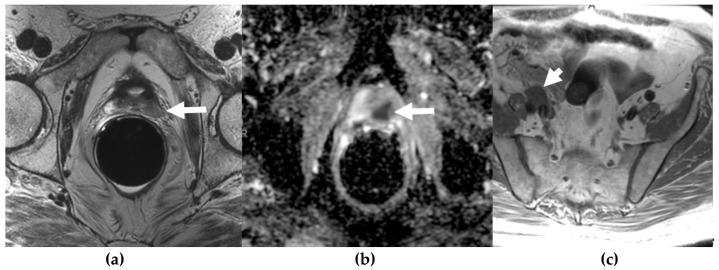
A 60-year-old man with lower retroperitoneal lymphadenopathy on PET-CT, found during work-up for dermatomyositis and abnormal digital rectal exam. T2W (a) and ADC (b) MR images through the prostate show a hypointense mass (long arrow) with restricted diffusion in the left posterolateral peripheral zone of the prostatic apex. Large field of view axial T1W MR image (c) of the pelvis shows right external iliac lymphadenopathy (short arrow). This was biopsy-proven metastatic small cell carcinoma of the prostate.
